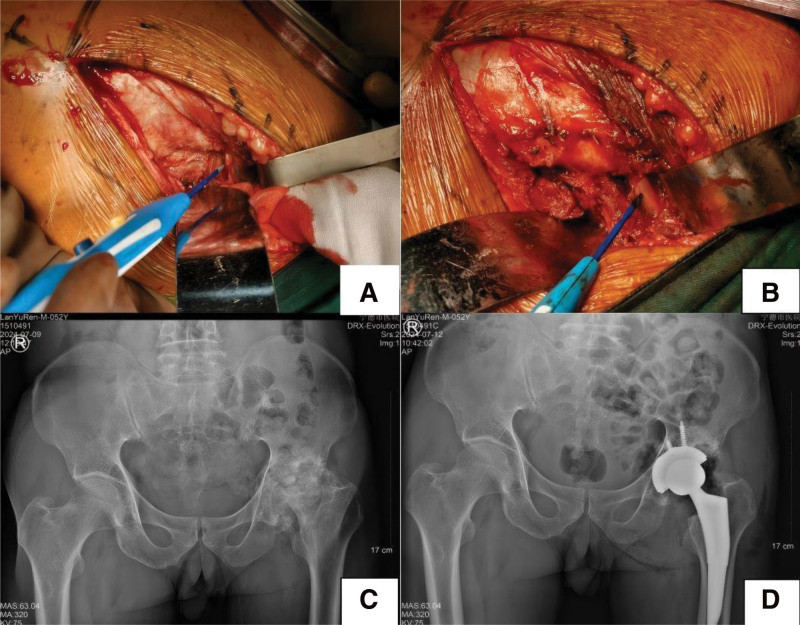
Male patient, 52 yr old, left DDH with OA, underwent total artificial replacement without cutting the piriformis muscle through the posterolateral approach. (A) The complete piriformis muscle was exposed during the operation. (B) The piriformis muscle was not severed during the operation. (C) Preoperative anteroposterior pelvic X-ray; (D) Postoperative anteroposterior X-ray of the pelvis. DDH = developmental dysplasia of the hip, OA = osteoarthritis.
